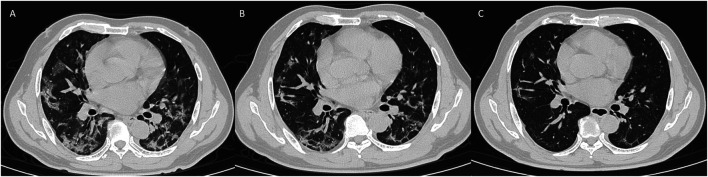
CT signs of a 47-year-old male on day 1 (A), day 5 (B), and day 17 (C) showed bilateral ground-glass opacity absorbed and fibrosis formation.
